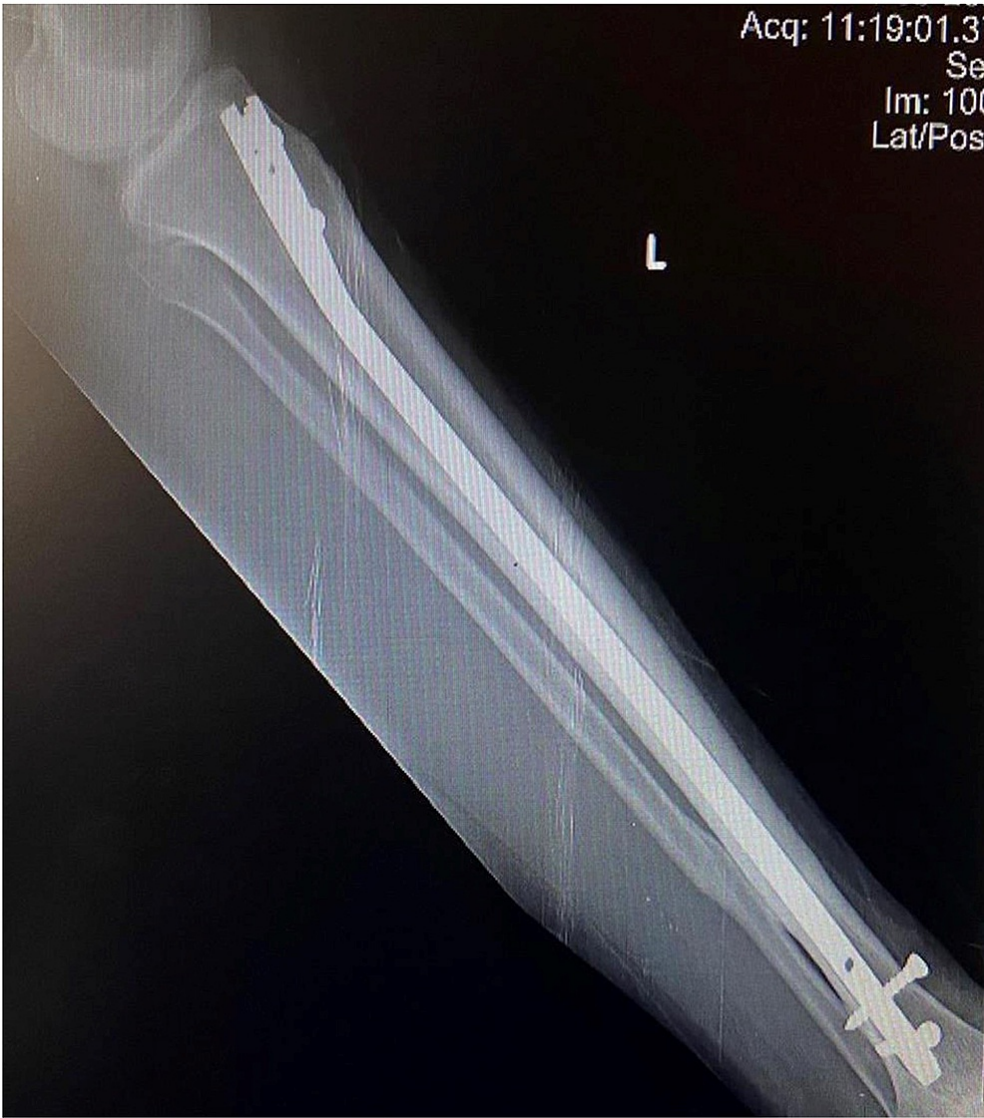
Six months follow-up lateral radiographs of a 34-year-old nonsmoker showing united distal third fracture of the left tibia with IMIL nail in situ
